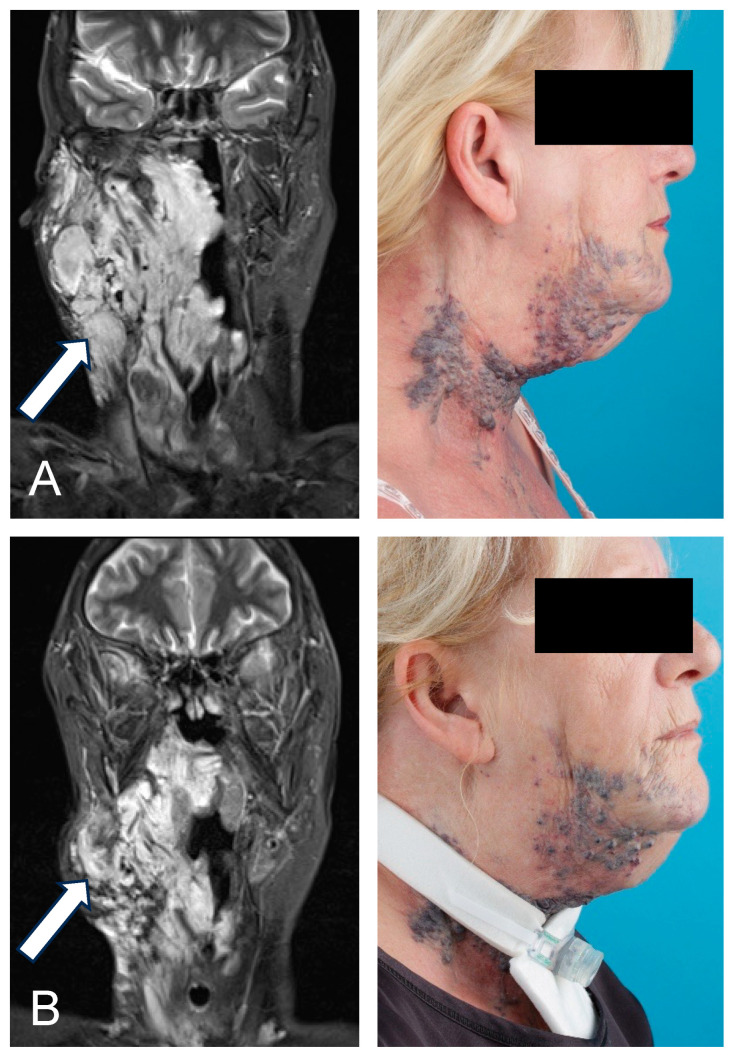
A 64-year-old female patient with an extensive venous malformation (VM, indicated by arrows) of the laryngeal and hypopharyngeal mucosa with severe bleeding, swelling, and dyspnea following bleomycin electrosclerotherapy (BEST). (A) T2-weighted (T2w) coronal magnetic resonance (MR) image and sagittal photographic documentation of the lesion prior to treatment, showing the extent of the VM. The patient reported symptoms of dysphagia and dyspnea. (B) T2w coronal MR image and sagittal photographic documentation taken 2 months after the first BEST session demonstrated regression of the lesion. However, postinterventional swelling and bleeding necessitated a tracheostomy, precluding a second BEST procedure.
